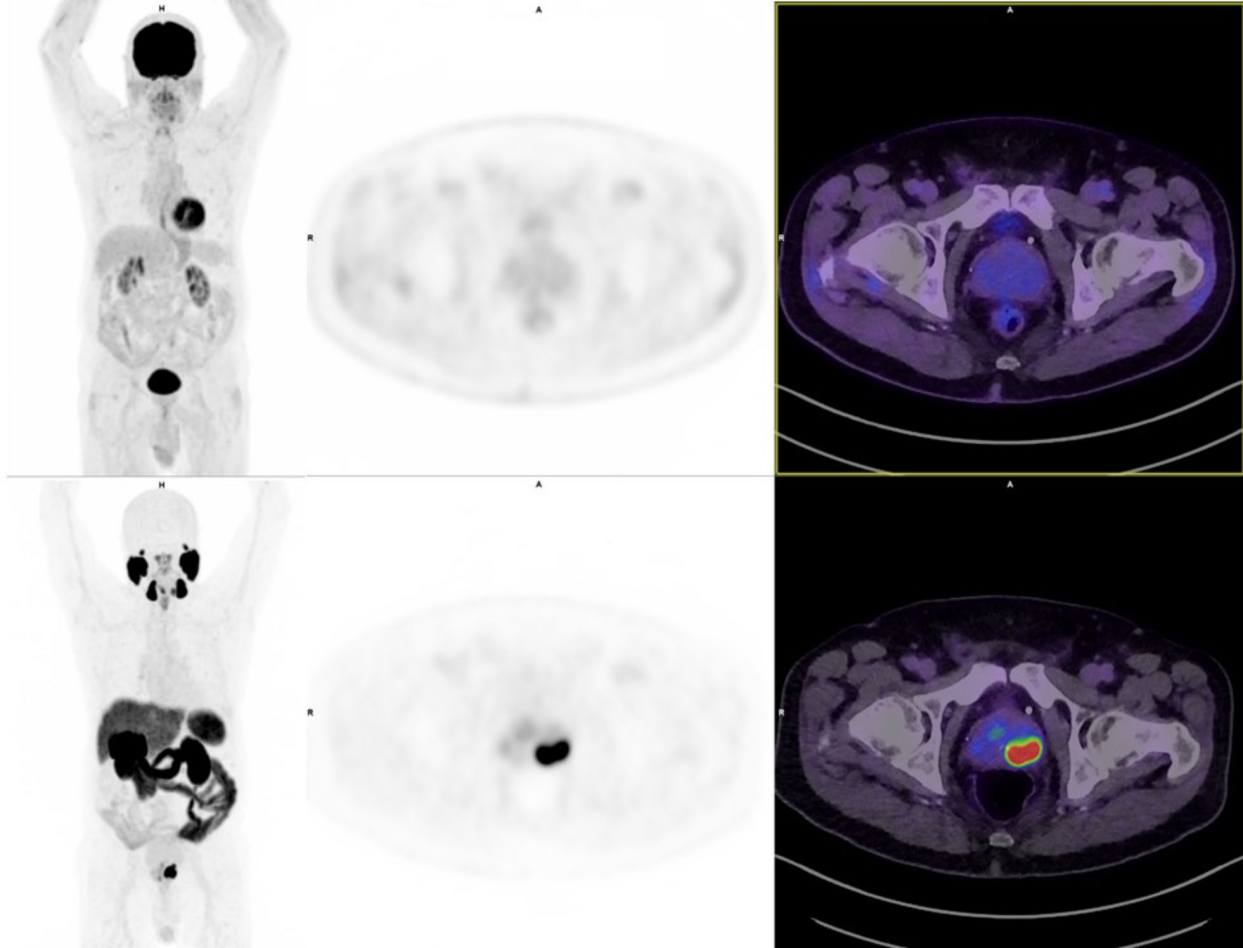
Intra-individual comparison of discordant imaging results with no uptake on [18F]FDG PET (upper row, left to right maximum intensity projection, axial PET and axial fused PET/CT, SUV window 0–10) and intense avidity on [18F]PSMA-1007 PET (lower row, SUV window 0–15) for a patient with Gleason score 4 + 5 = 9, stage pT3b prostate cancer
